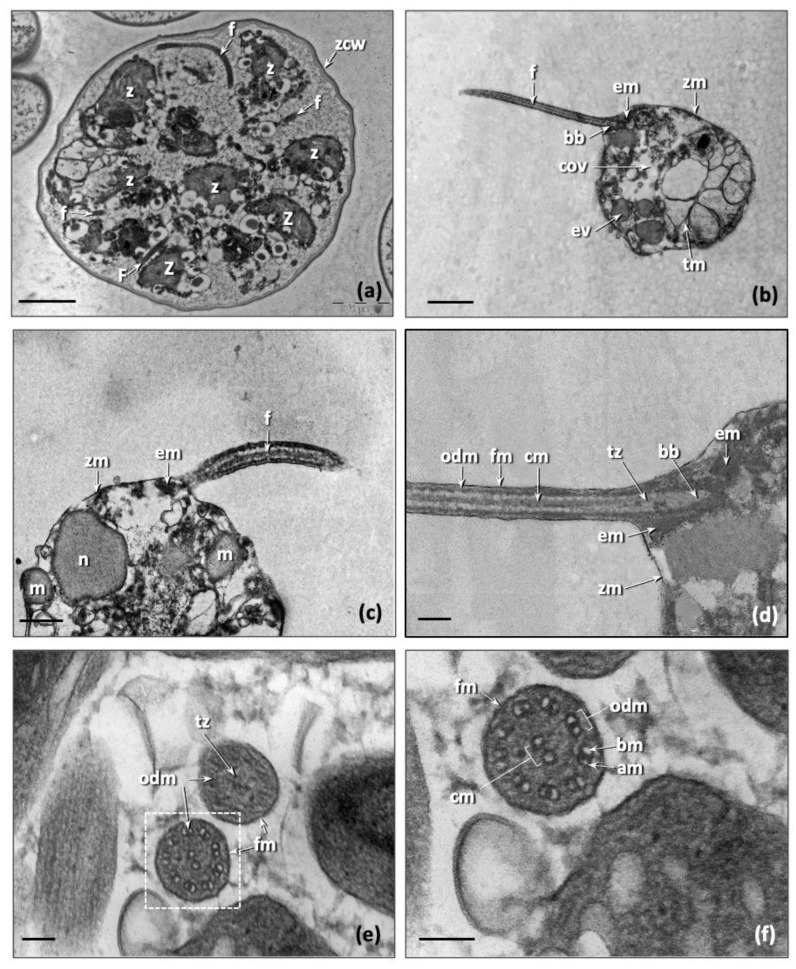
The zoospores of *Trebouxia lynnae* sp. nov.: (a) zoosporangium with several zoospores; (b,c) longitudinal section of a zoospore; (d) longitudinal view of the flagella insertion in the zoospore; (e) cross section of two flagella in a young zoospore, one in the filament zone and another in the transition zone; (f) detail of the filament zone flagella. Abbreviations: am (microtubule a), bb (basal body), bm (microtubule b), cm (central microtubules), cov (contractile vesicle), em (electrodense material), ev (electrondense vesicle), f (flagellum), fm (flagellum membrane), m (mitochondria), n (nucleus), odm (outer microtubules doublet), tm (thylakoidal membrane), tr (transition region), tz (transition zone), zcw (zoosporangium cell wall), z (zoospore), zm (zoospore membrane). White arrows point to the peculiar ultrastructures identified by the abbreviations. Scale bars: (a) =2 μm, (b) =1 μm, (c) =400 nm, (d) =200 nm, (e,f) =100 nm. Reference strain: ASUV 44.
